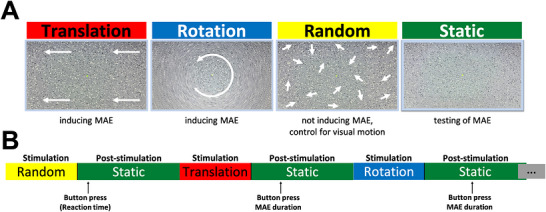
(A) Visual dot motion stimulation conditions used in the experiment (black and white dots on a gray background, green fixation dot at the center). The direction of motion is indicated by white arrows. (B) Excerpts of a typical MRI timeline including the different visual motion conditions (translation, rotation, and random) applied in pseudorandomized order, each followed by the static dot pattern. Exemplary button press times indicating the end of perceived MAE (self‐motion) are given.
